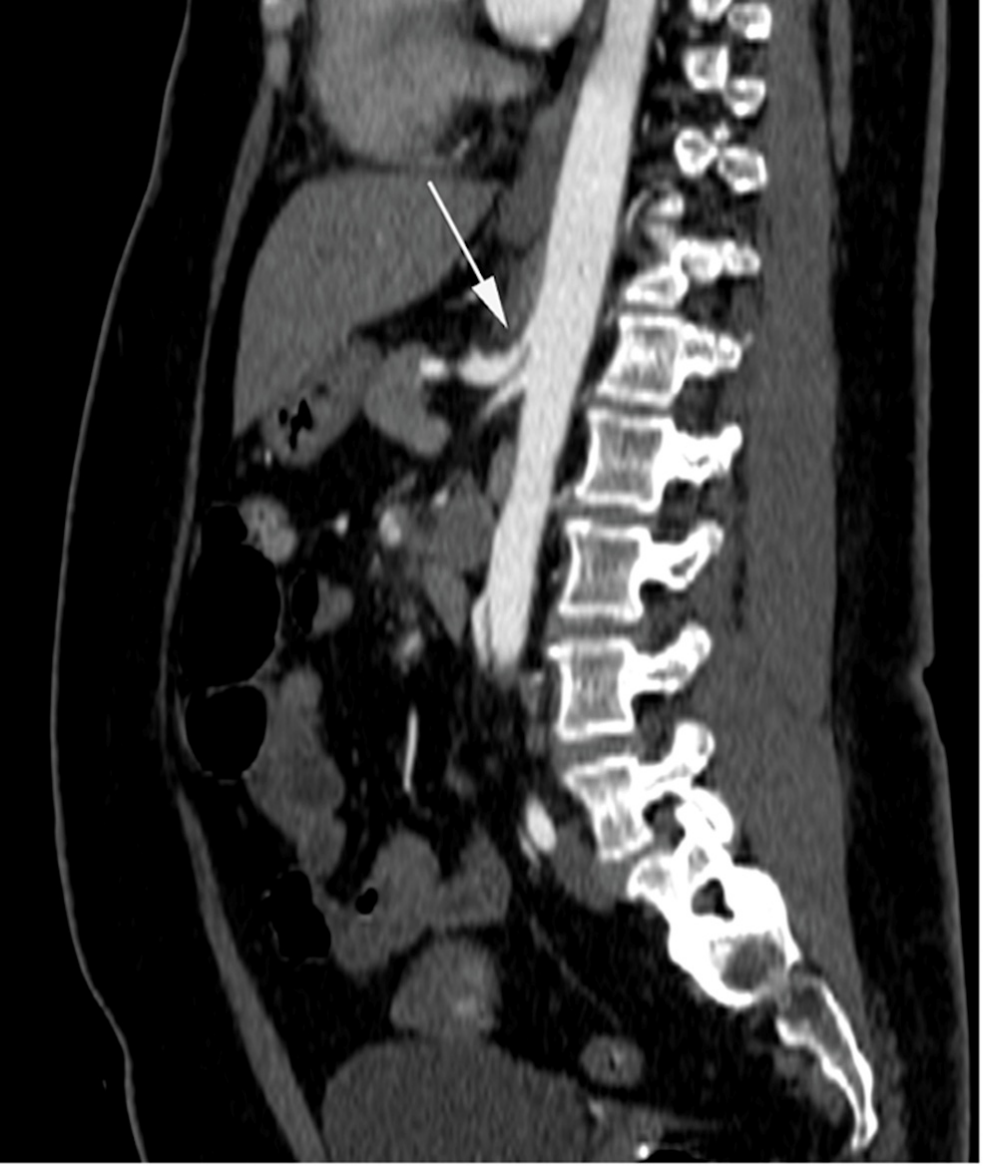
Sagittal reformatted, post intravenous contrast computed tomography White arrow demonstrates short segment stenosis of the coeliac trunk with a characteristic hooked appearance and post-stenotic dilatation.
